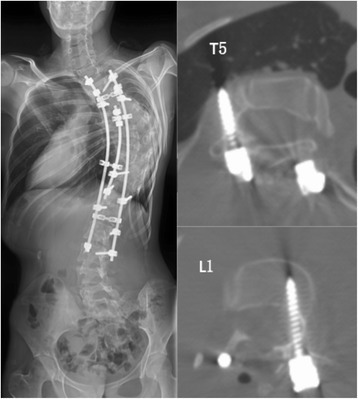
Postoperative CT scanning demonstrating lateral perforation in T5 level and medial perforation in L1 level
